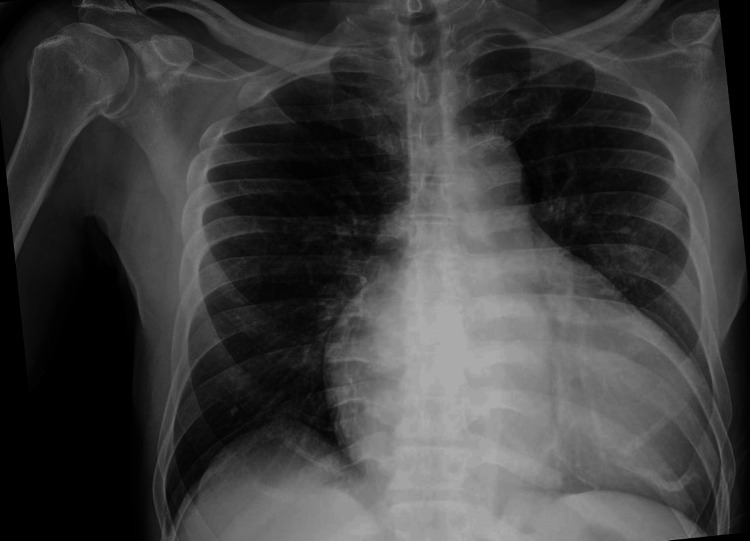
The patient's preoperative chest x-ray, which shows an enlarged cardiomediastinum.
